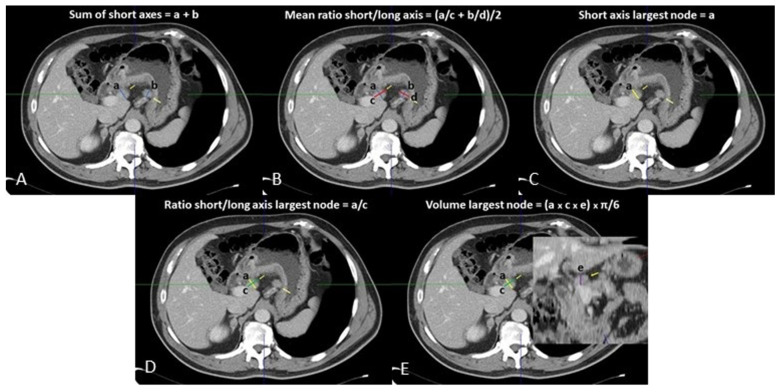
Loco-regional CT measurements: (A) sum of short axes; (B) mean of ratios of short/long axes; (C) short axis of largest lymph node; (D) ratio of short/long axis of largest lymph node; (E) volume of largest lymph node.
